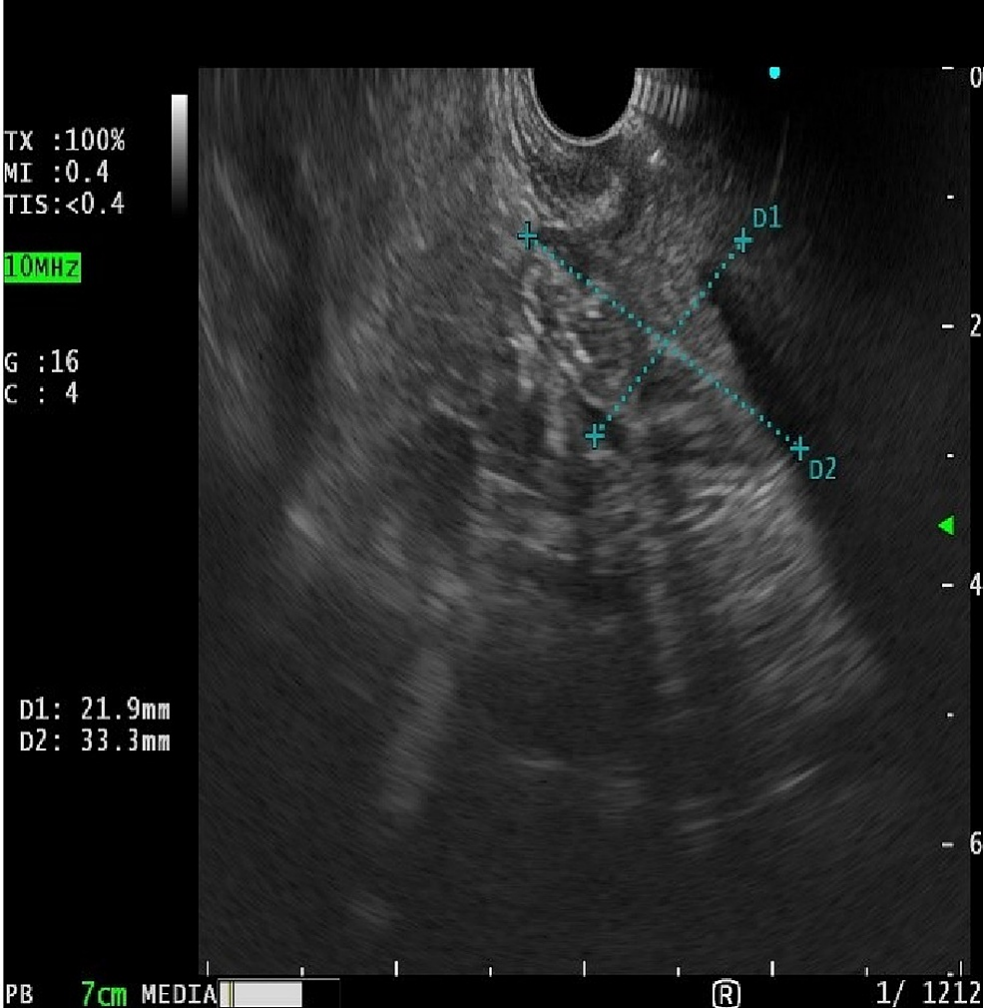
Endoscopic ultrasonography (EUS) revealed tumor mass originating from the second ultrasound layer with heterogenous isoechoic structure.
